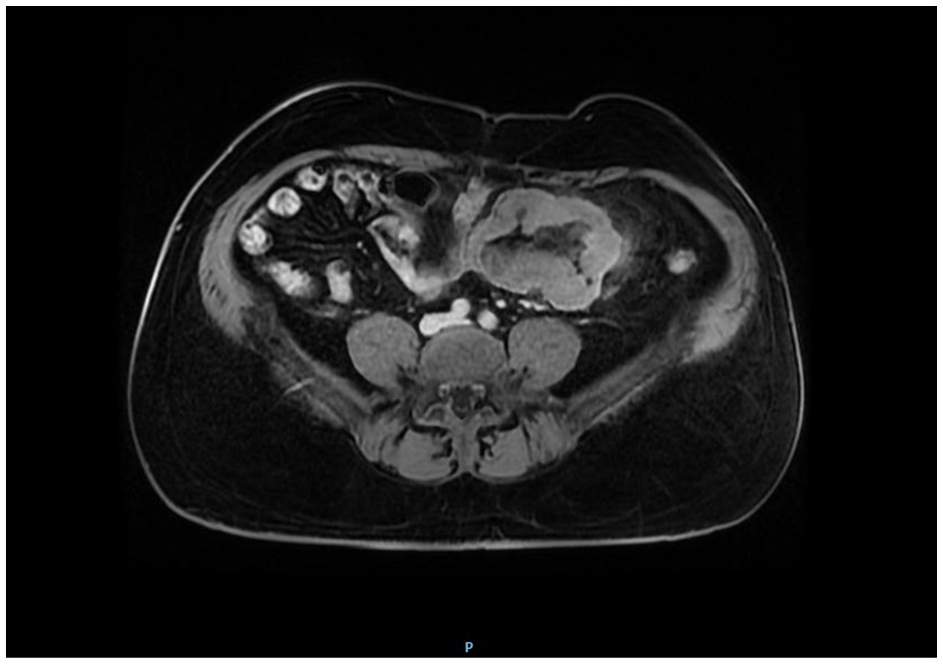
Low intensity on T1-weighted imaging. On the axial magnetic resonance image, the tumor showed an intestinal involvement due to lymphoproliferative disease.
